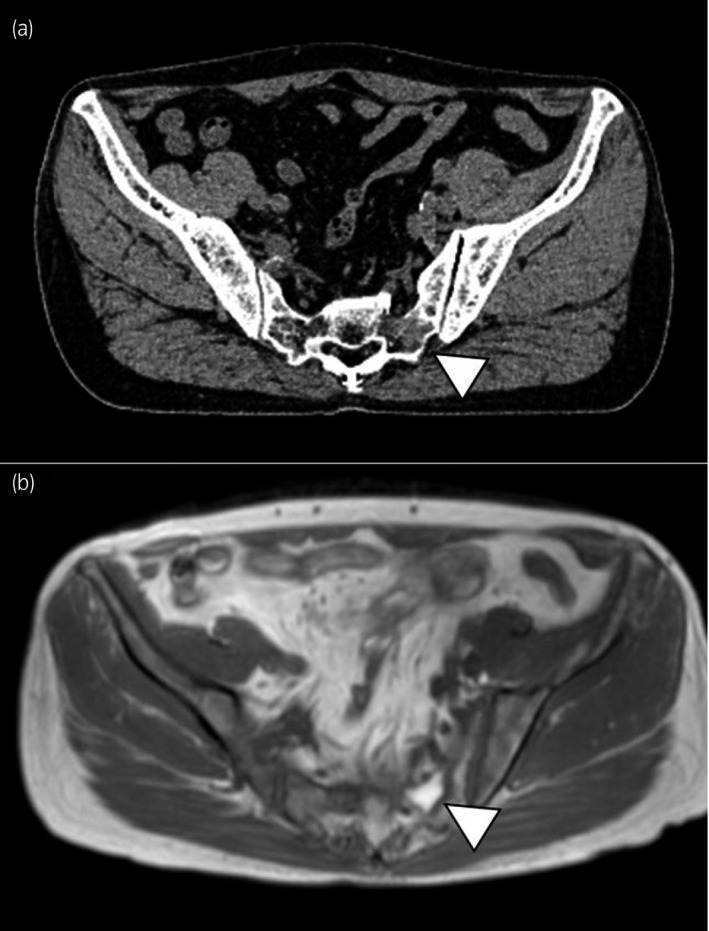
A 75‐year‐old man with BCR after RP in 2008 and SRT in 2013. In 2017, his PSA level increased to 0.44 ng/mL. Osteolytic bone metastasis in the sacrum cannot be detected with CT (a), without the results of DWIBS (b).
